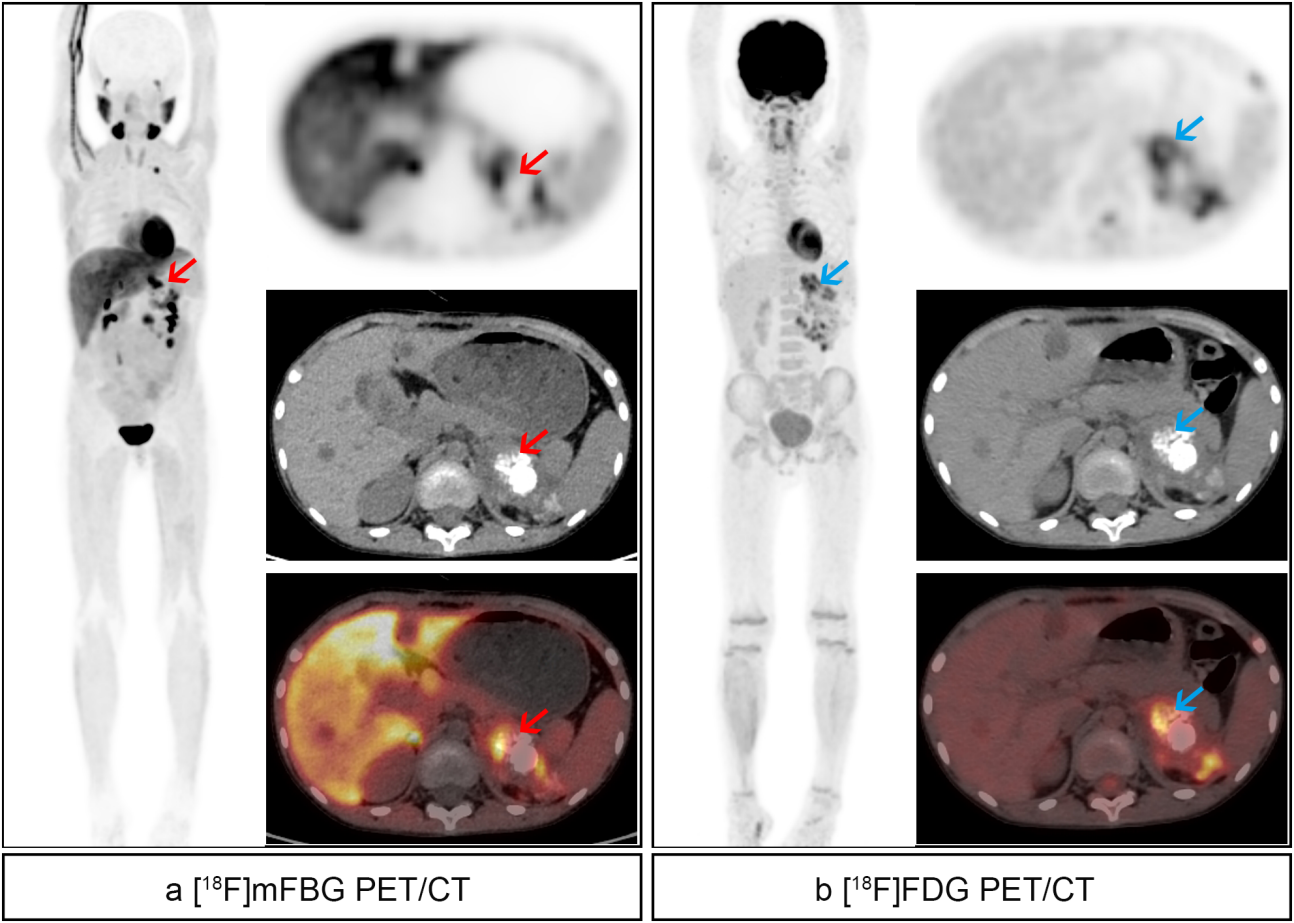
An 8-year-old boy with high-risk left retroperitoneal neuroblastoma received 1 cycle of chemotherapy. (a) [^18^F]mFBG images. Pathological uptake (red arrows) with SUVmax 8.9 and TBR 12.5 was noted in the left retroperitoneal mass, and detailed showed in axial images. (b) [^18^F]FDG images. Pathological uptake (blue arrows) with SUVmax 5.8 and TBR 11.9 was noted in the left retroperitoneal mass, and detailed show in axial images. Besides, residual activity of mFBG and FDG was found in the left thoracic entrance and retroperitoneal lymph nodes.
